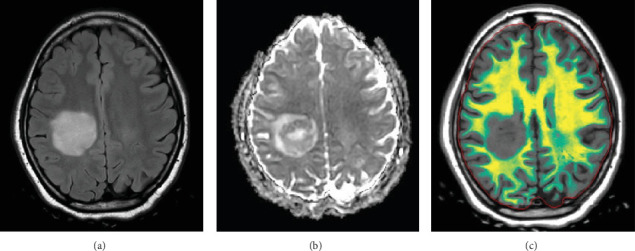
Axial images from brain MRI (3 weeks after admission) demonstrating a hyperintense lesion on (a) T2 FLAIR in the right centrum semiovale and corona radiata measuring 3.7 × 3.7 cm. The lesion demonstrates decreased ADC values (b) in keeping with reduced diffusivity. Synthetic MRI myelin map (c) demonstrates a “ring of decreased myelination” around the lesion.
